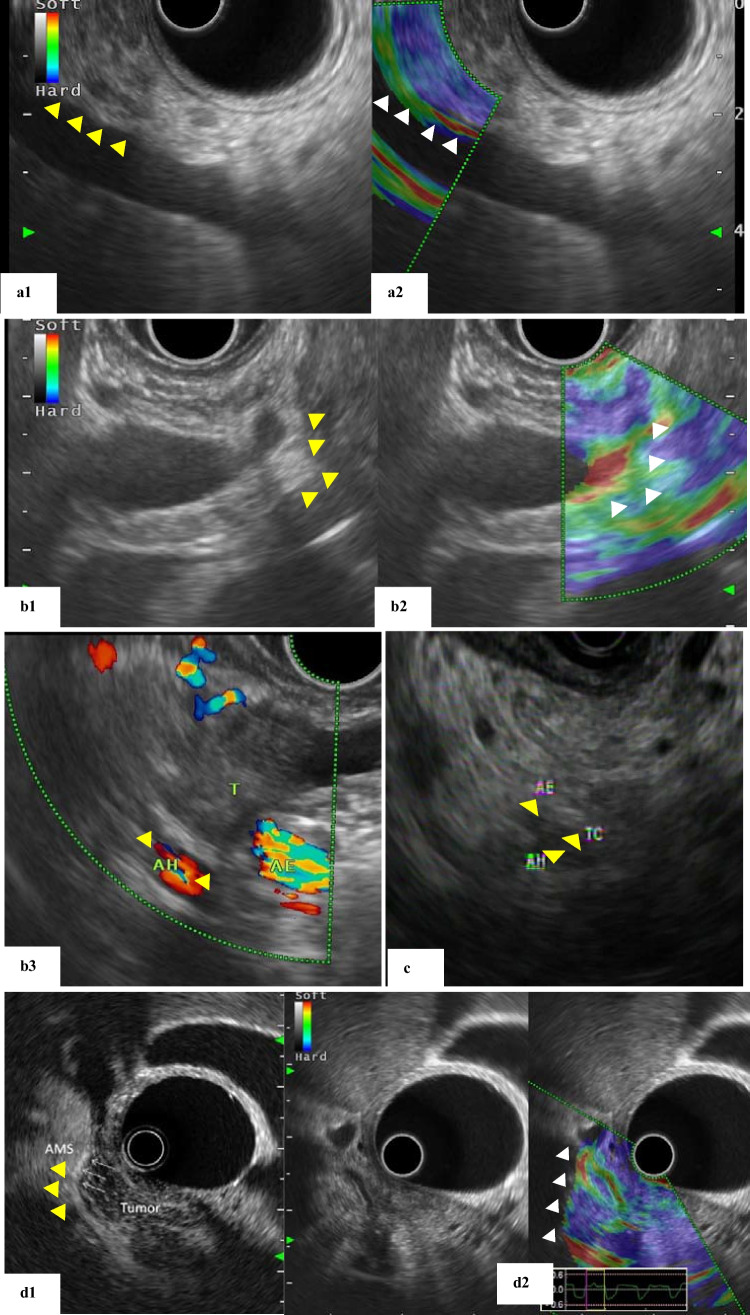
Diagnosis of disruption of the plane III of Inoue by endoscopic ultrasonography and elastography (EUS-EG). a1, b1, c, and d1 EUS-B mode image with hyperechoic line with no disruption (yellow arrows). a2, b2, and d3 EUS-EG image clearly depicts no disrupted plane and green line-low echo area (white arrows). b3 EUS-B Doppler mode with hyperechoic plane and no VI margin. d2 EUS-B mode with unclear tumour involvement
